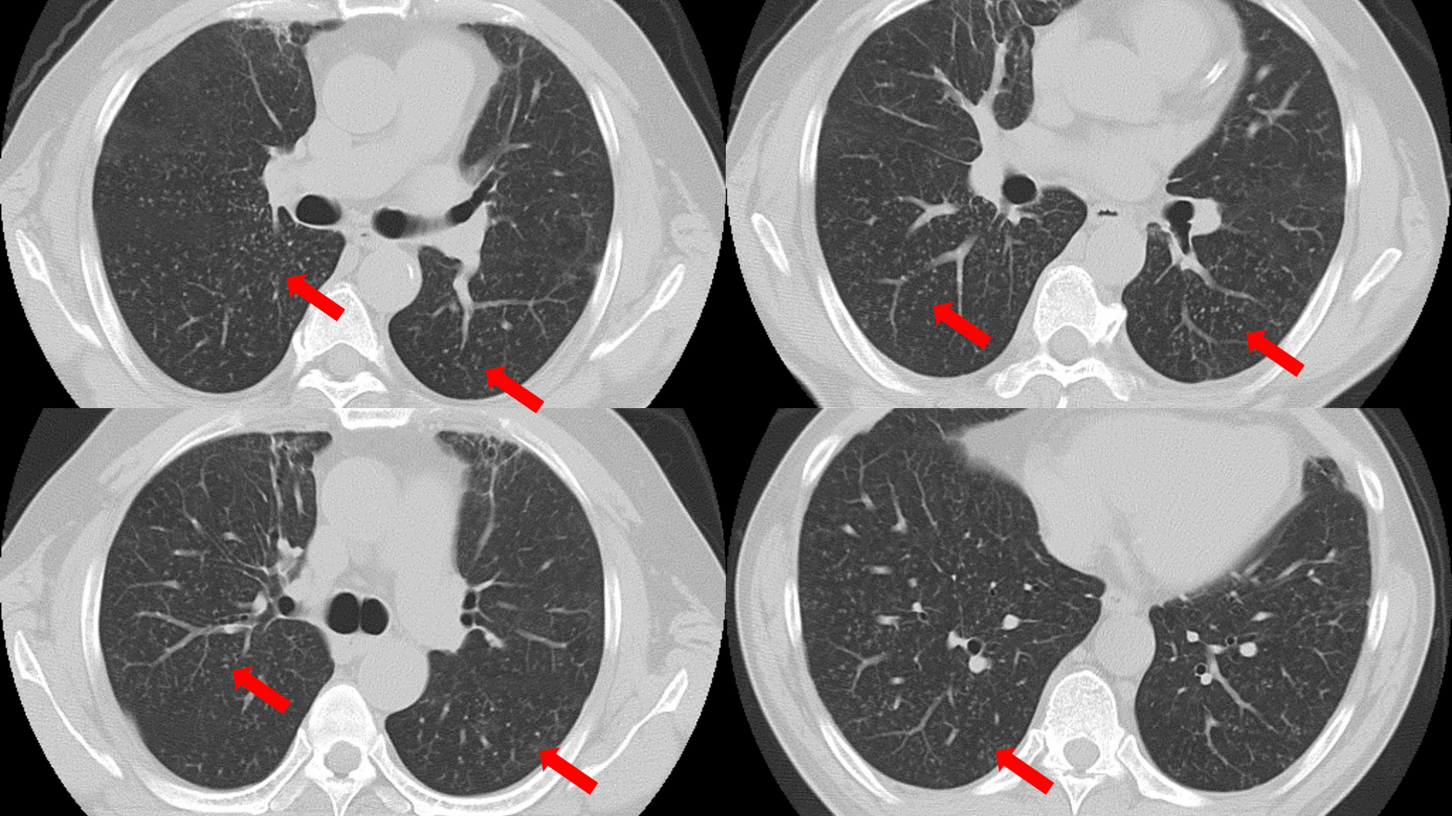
Axial chest computed tomography image one year later The diffuse infiltrative shadow had disappeared, leaving only a slight miliary shadow along the microbronchi.
